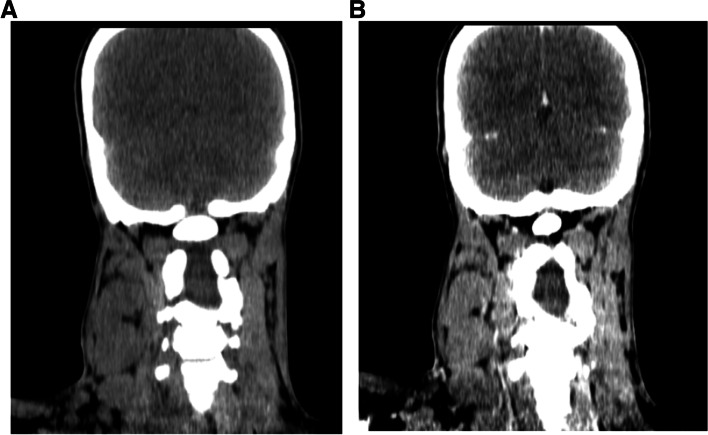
A An unenhanced coronal CT of the head and neck. There were multiple enlarged right level II, III, IV and V lymph nodes measuring up to 6 cm. B A contrast enhanced coronal CT, which demonstrated poorly enhancing right level II, III, IV and V lymph nodes. A diagnosis of lymphoma was made on both A and B
